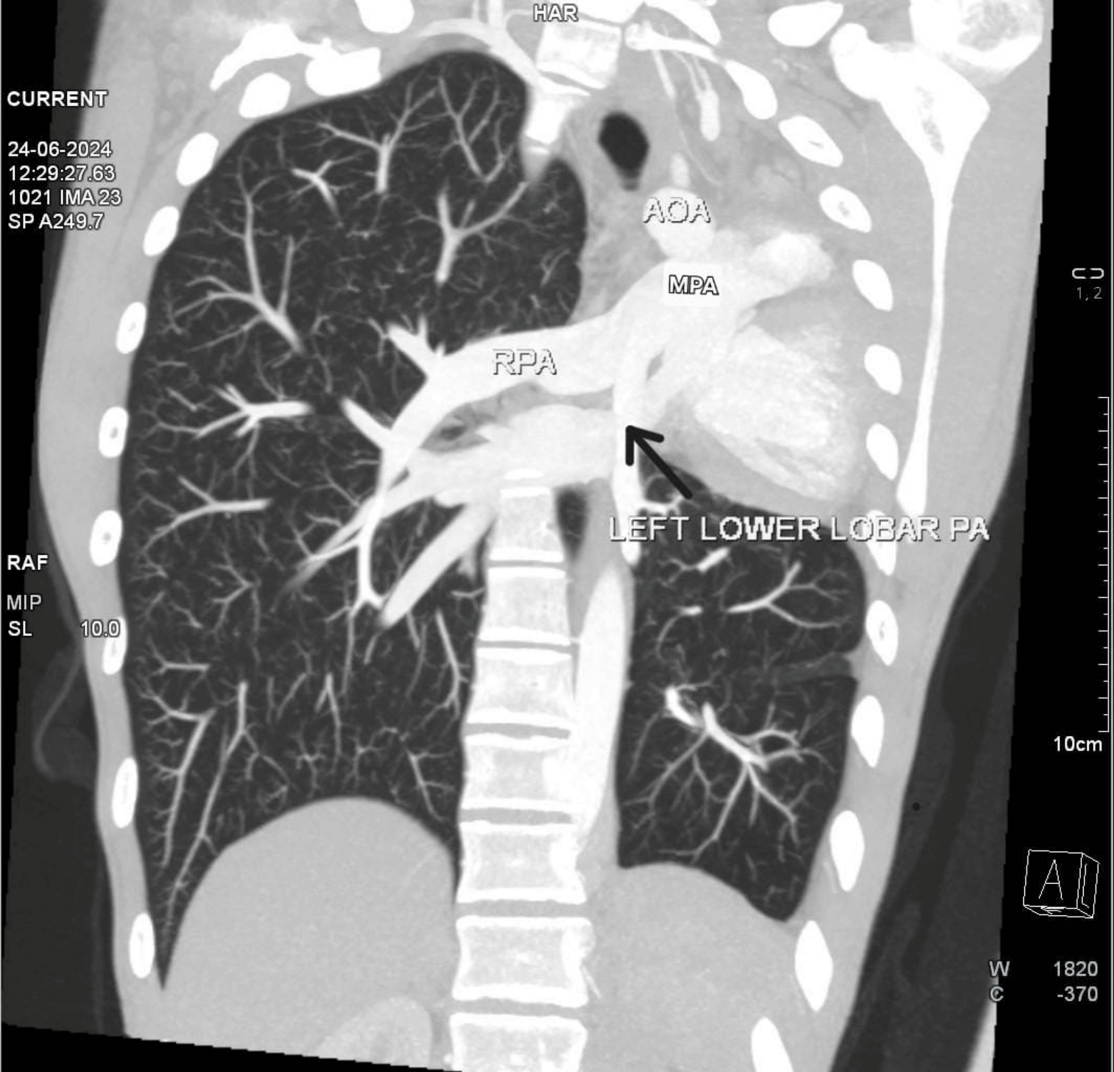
CT pulmonary angiogram CT pulmonary angiogram is suggestive of displaced heart and great vessels in empty space due to the absent left upper lobe. The image shows the left inferior lobar pulmonary artery branching from the main pulmonary artery (black arrow) CT: computed tomography
